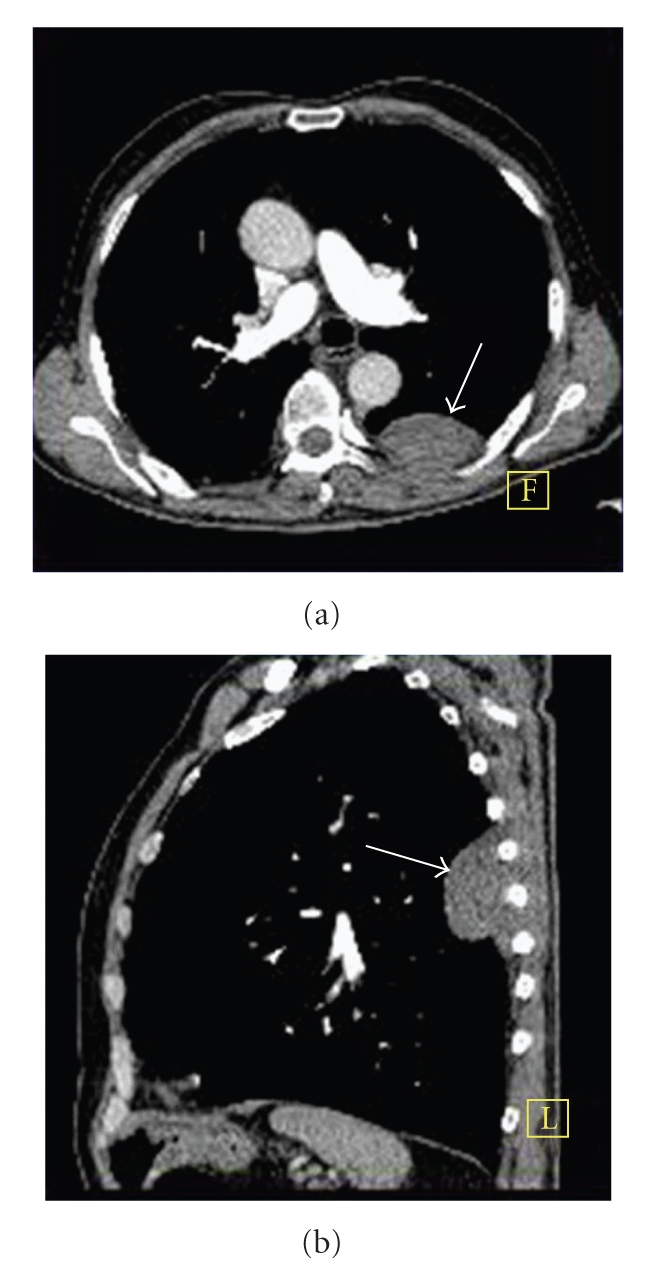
Axial (a) and sagittal (b) CT images demonstrate a lesion in the upper lobe of the left lung invading pleura and adjacent ribs (arrows).
